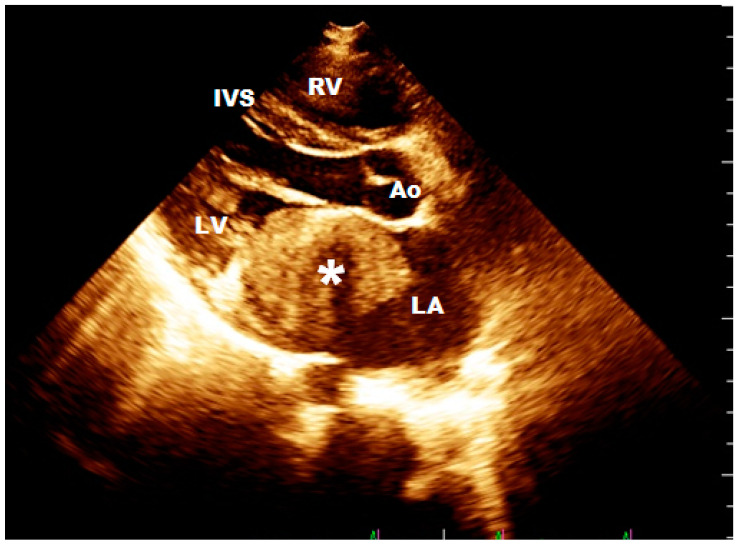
Two-dimensional echocardiography: parasternal long axis image obtained in a 16-year boy shows a huge hyperechoic mass (*) in the left atrium (LA) which was significantly hindering the flow of blood to the left ventricle through the mitral valve. RV—Right Ventricle; IVS—Interventricular Septum; LV— Left Ventricle; LA—Left Atrium; Ao—Aorta.
